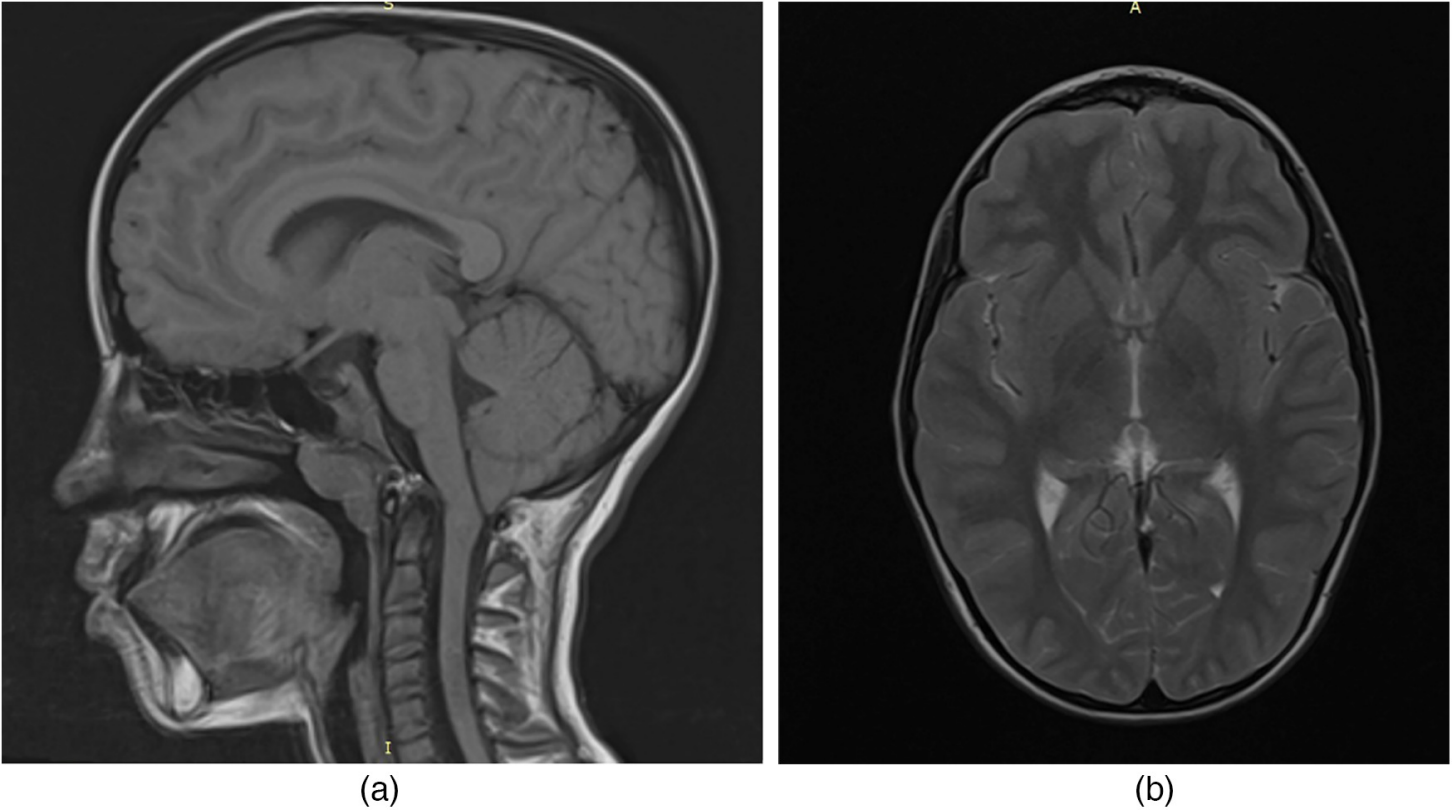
MRI of the child's brain with acid sphingomyelinase deficiency (ASMD) type A/B disease and Arnold-Chiari I malfunction: (a) MRI section in the sagittal plane; (b) MRI section in the axial plane.
